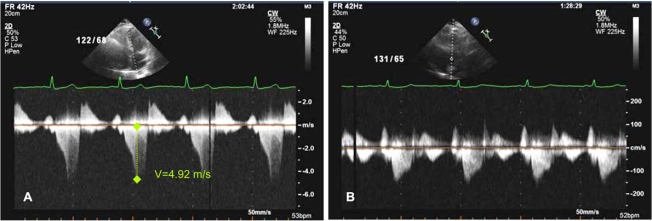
Transthoracic echocardiogram in hypertrophic obstructive cardiomyopathy: continous wave Doppler across the LVOT (A) before ASA indicating severe obstruction with a dynamic gradient of 96 mmHg and (B) 6 months after ASA with resolution of the obstruction.
